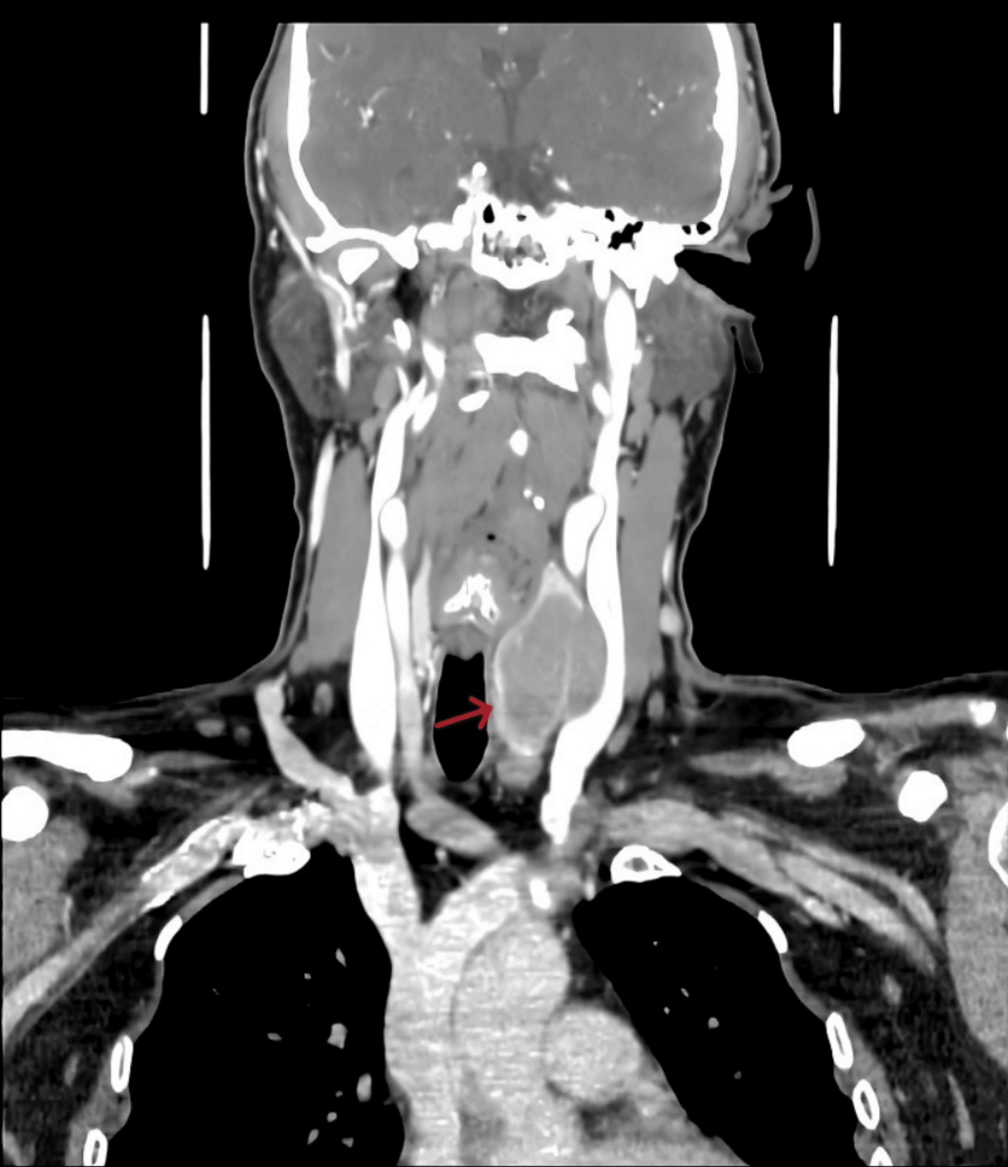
CECT neck coronal section showing a well-defined lobulated exophytic soft tissue lesion involving the left lobe and isthmus of the thyroid (red arrow) CECT: Contrast-enhanced computed tomography.
